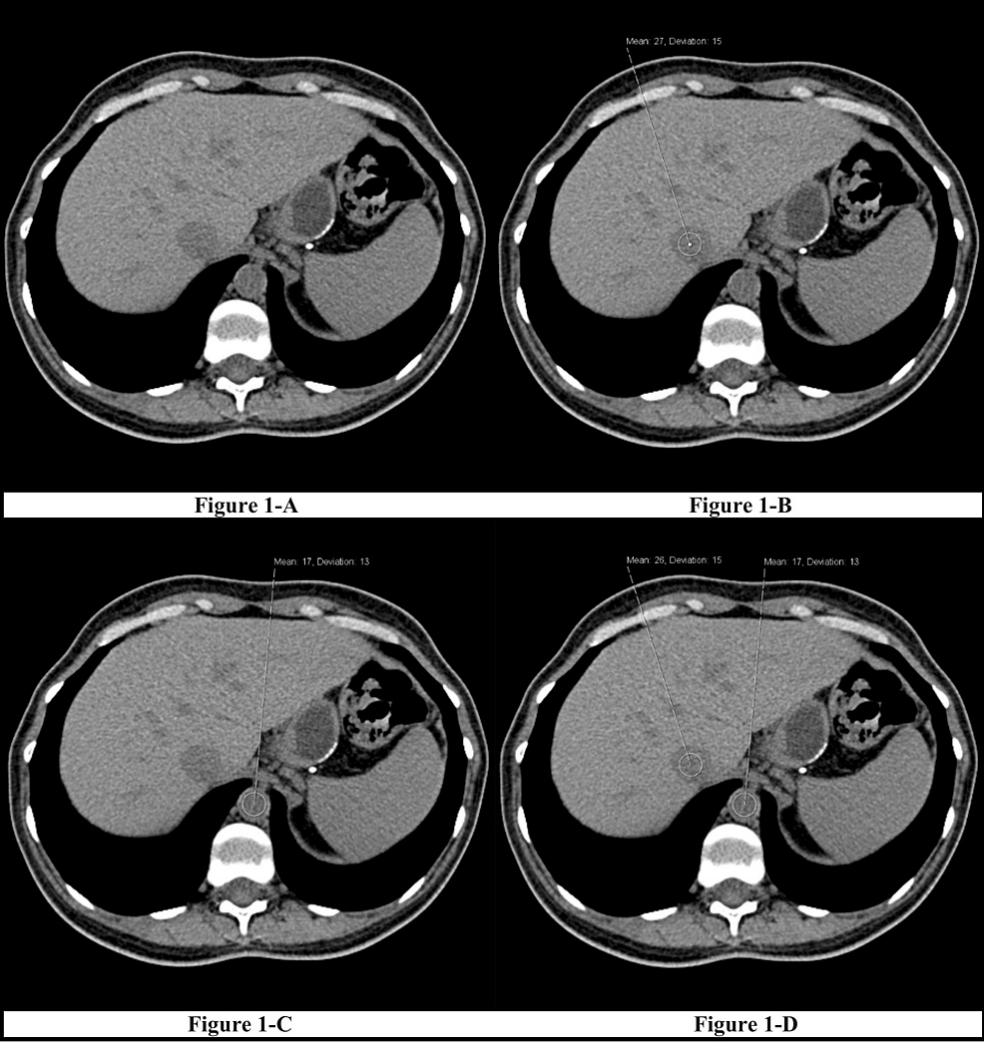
An example of axial CT images with measurements at the IVC and the aorta 1A-1D: Collection of vessel densities for the CT scans; 1A: the general level of the CT scans taken; 1B: Measurement taken from the intrahepatic IVC; 1C: Measurement taken from the abdominal aorta; 1D: Final CT measurements for data collection IVC: inferior vena cava
